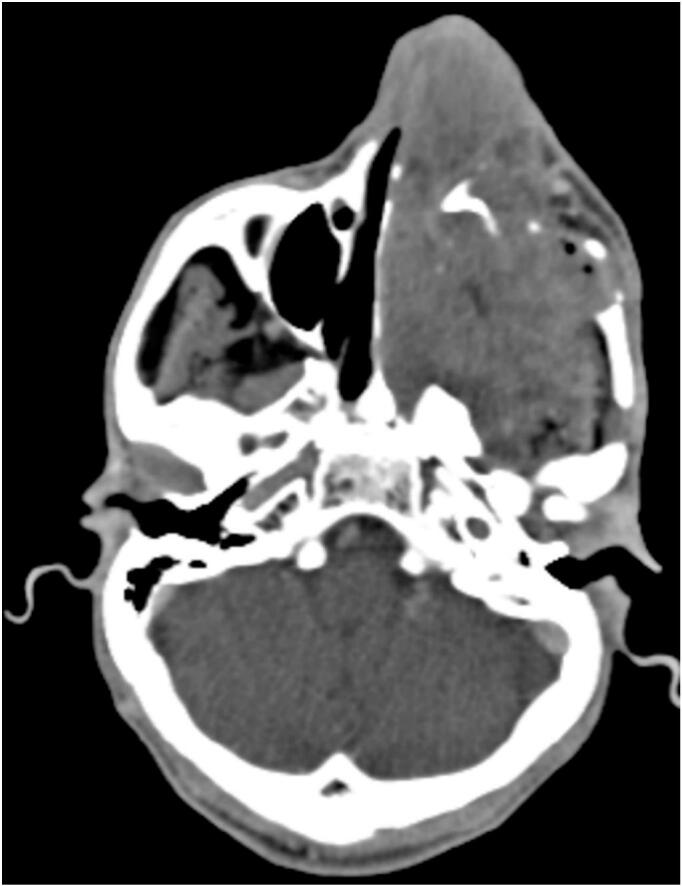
Maxillofacial CT scans showing an expansile radiolucent/radiopaque lesion, with lytic changes, located in the anterior left maxilla, extending superiorly into the left maxillary sinus.
